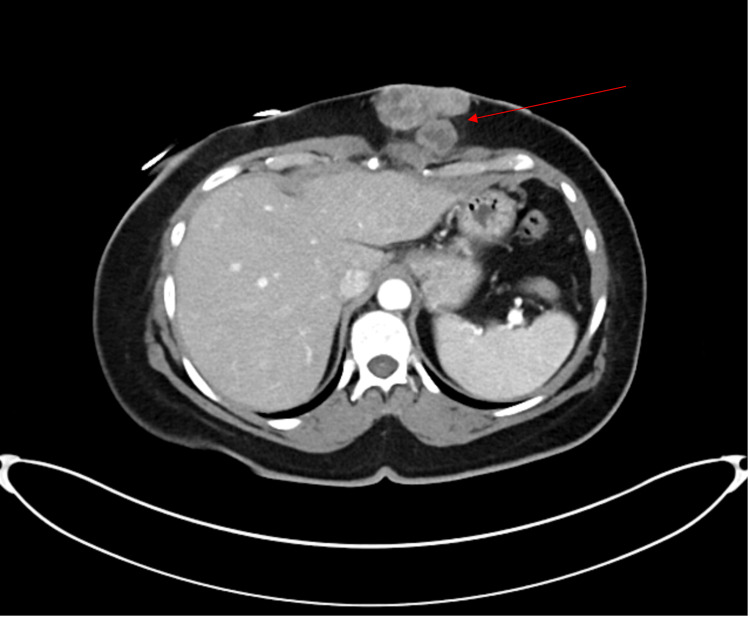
Axial contrast-enhanced CT scan of the abdomen and pelvis (CTAP) showing a 5.2 × 3.9 cm lobulated, heterogeneous soft tissue mass (red arrow) in the anterior abdominal wall, primarily within the subcutaneous fat. The mass abuts and possibly infiltrates the rectus muscle without deeper extension.
